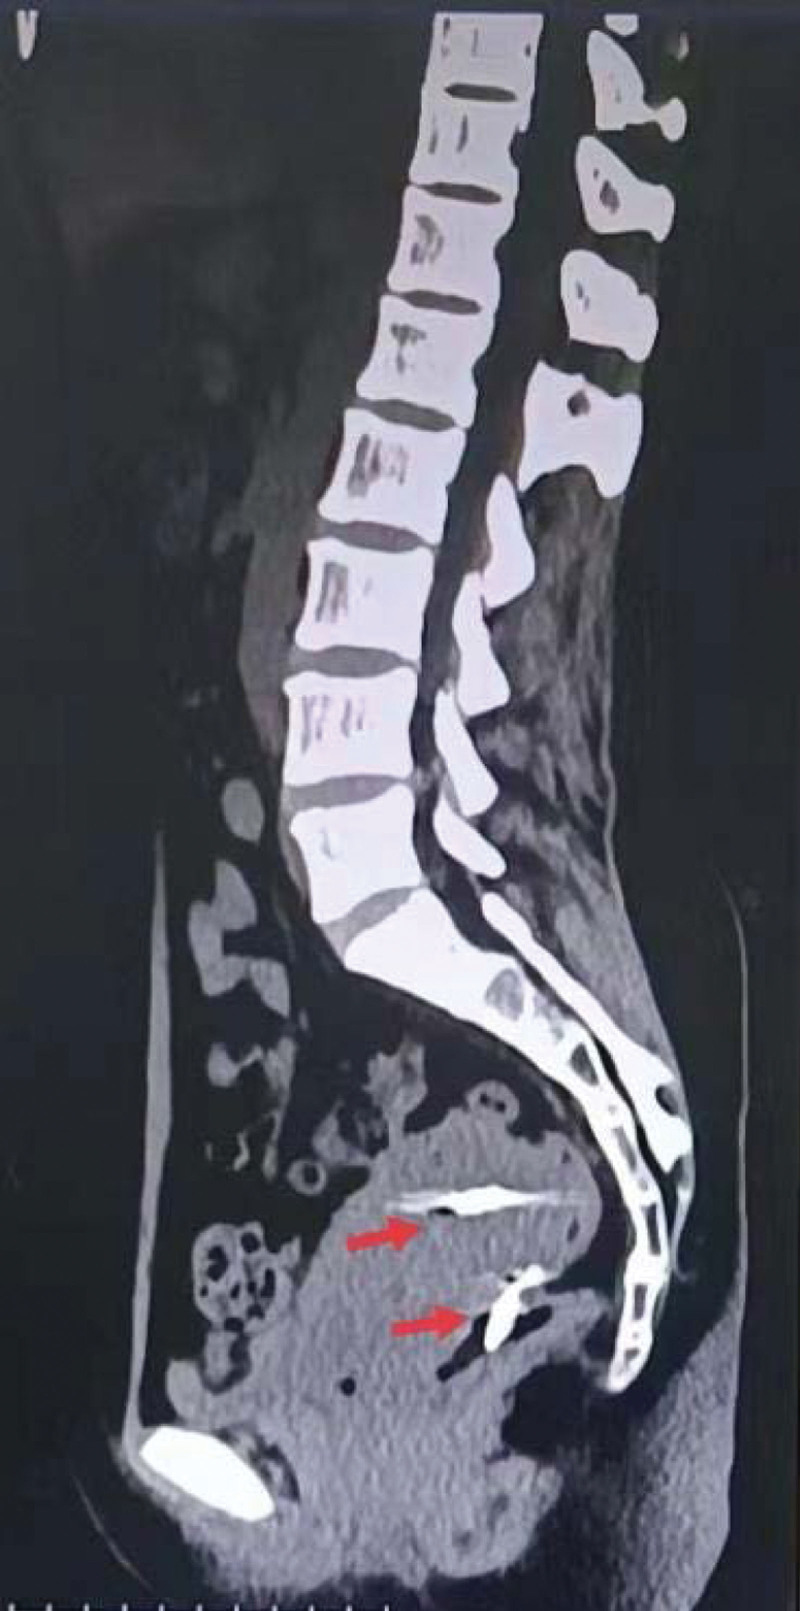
CT images of 2 intrauterine devices. One was intrauterine (indicated by the red arrow at the top), and the other penetrated into the intestinal cavity (indicated by the red arrow at the bottom). CT = computed tomography.
